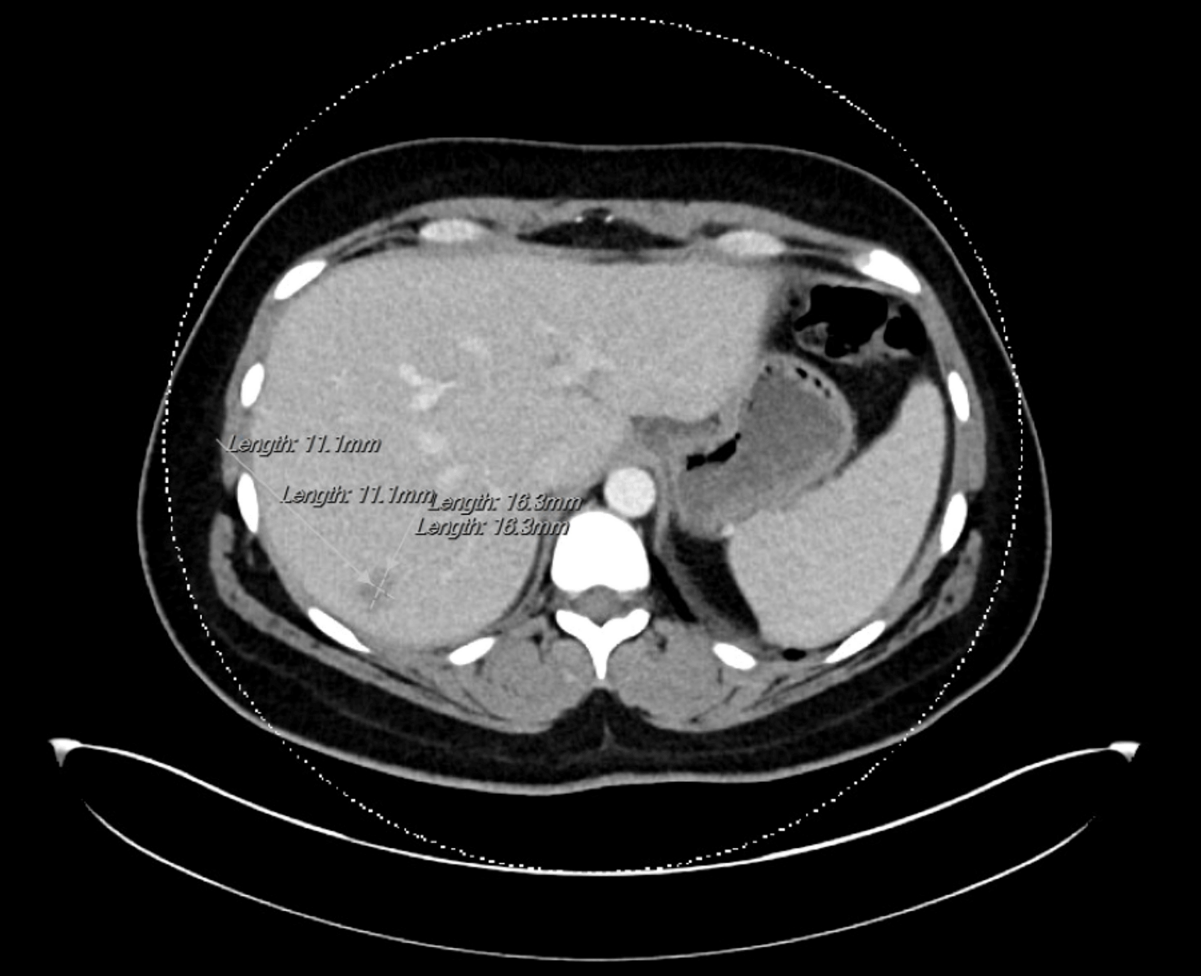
CT CAP with contrast showing a hepatic hypoattenuating lesion measuring 1.3 x 1.6 x 1.1 cm CAP: chest, abdomen, and pelvis; CT: computed tomography
